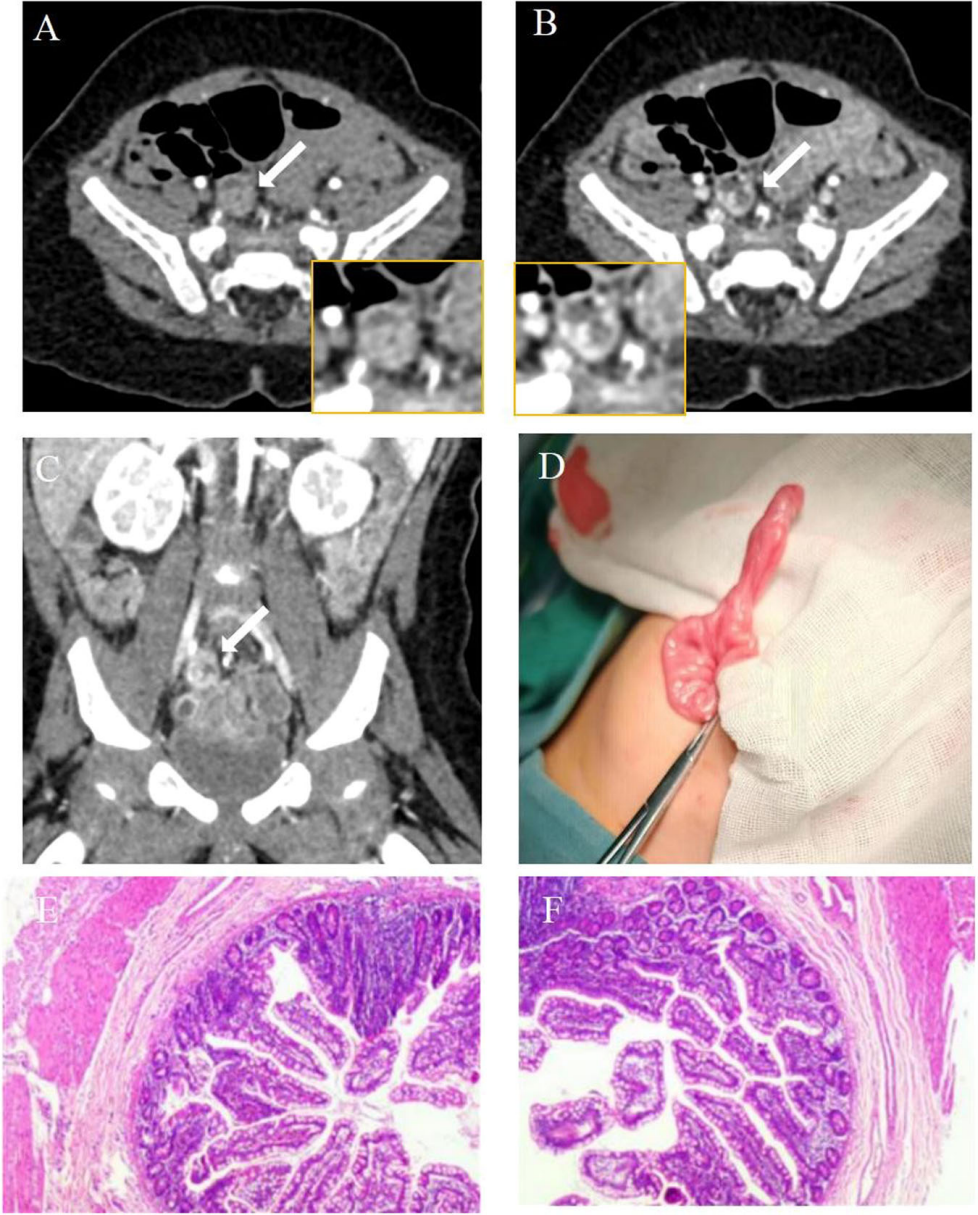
A 6-month-old boy was admitted to the hospital due to recurrent bloody stools, with a hemoglobin level of only 71 g/L. Following an inconclusive diagnosis from an abdominal ultrasound, an enhanced CT scan was subsequently performed, with Meckel’s diverticulum bleeding considered as a preliminary diagnosis. In the arterial (A) phase, the image revealed a sac-like diverticulum (as indicated by the arrow), while in the venous phase, the diverticulum gradually filled, observable in axial (B) and coronal (C) views (as indicated by the arrow). One day after the CT examination, a laparoscopic small intestine resection surgery was performed, and intraoperative images (D) showed a diverticular structure in a linear shape (located 35 cm from the ileocecal valve). Subsequent pathological examination (E, F) confirmed Meckel’s diverticulum (HE × 40, × 40)
